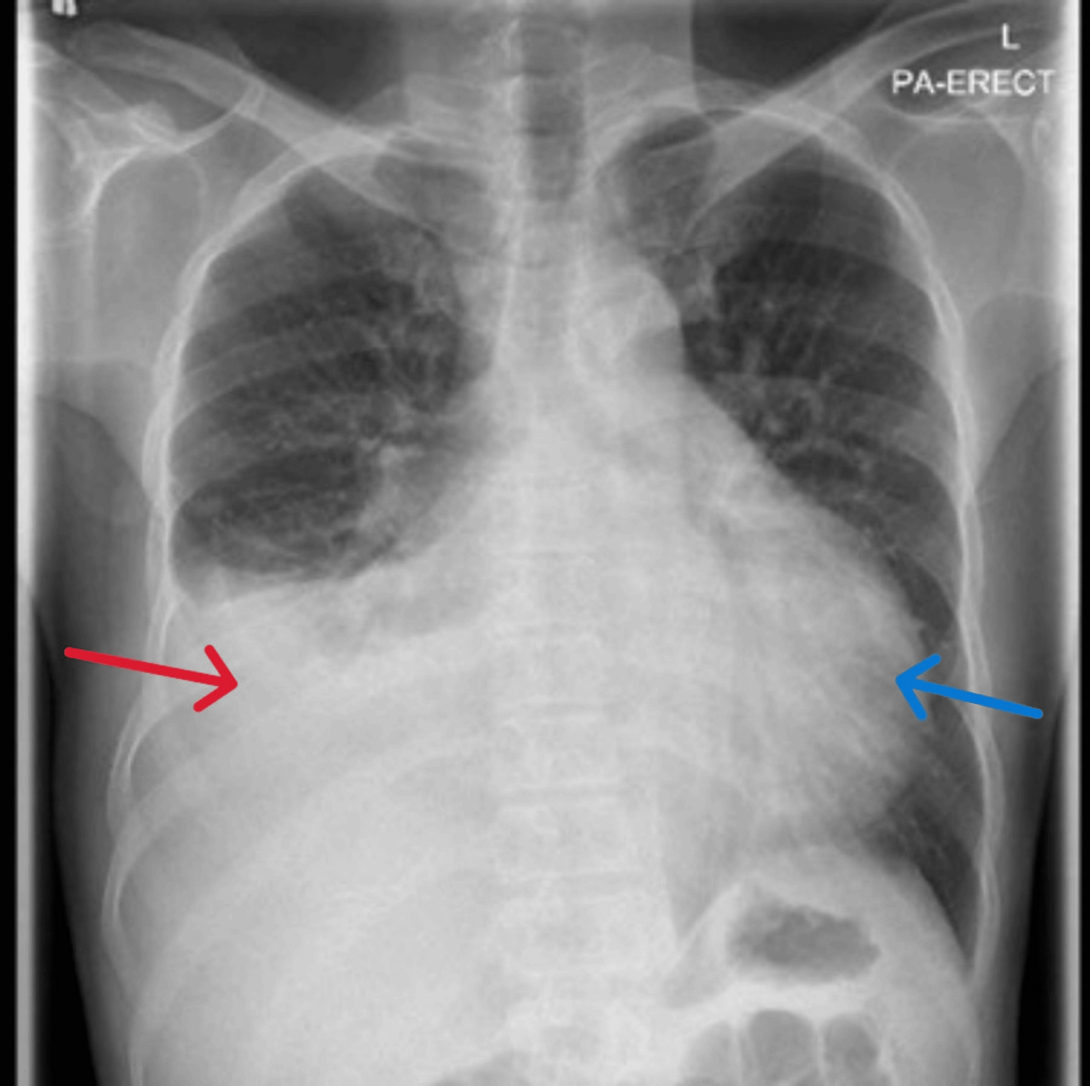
Chest X-ray showing moderate right pleural effusion (red arrow) and cardiomegaly (blue arrow).
